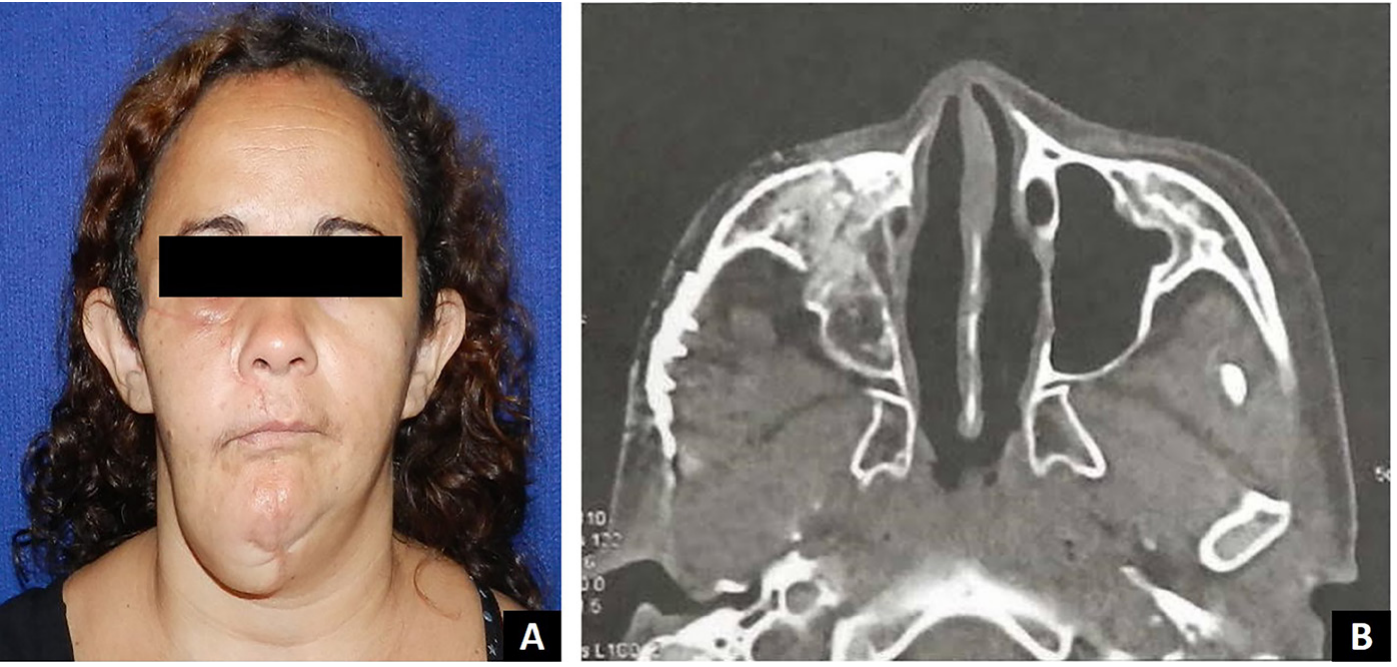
(A) Clinical follow-up of 36 months. (B) Axial CT scan of follow-up of 36 months.
